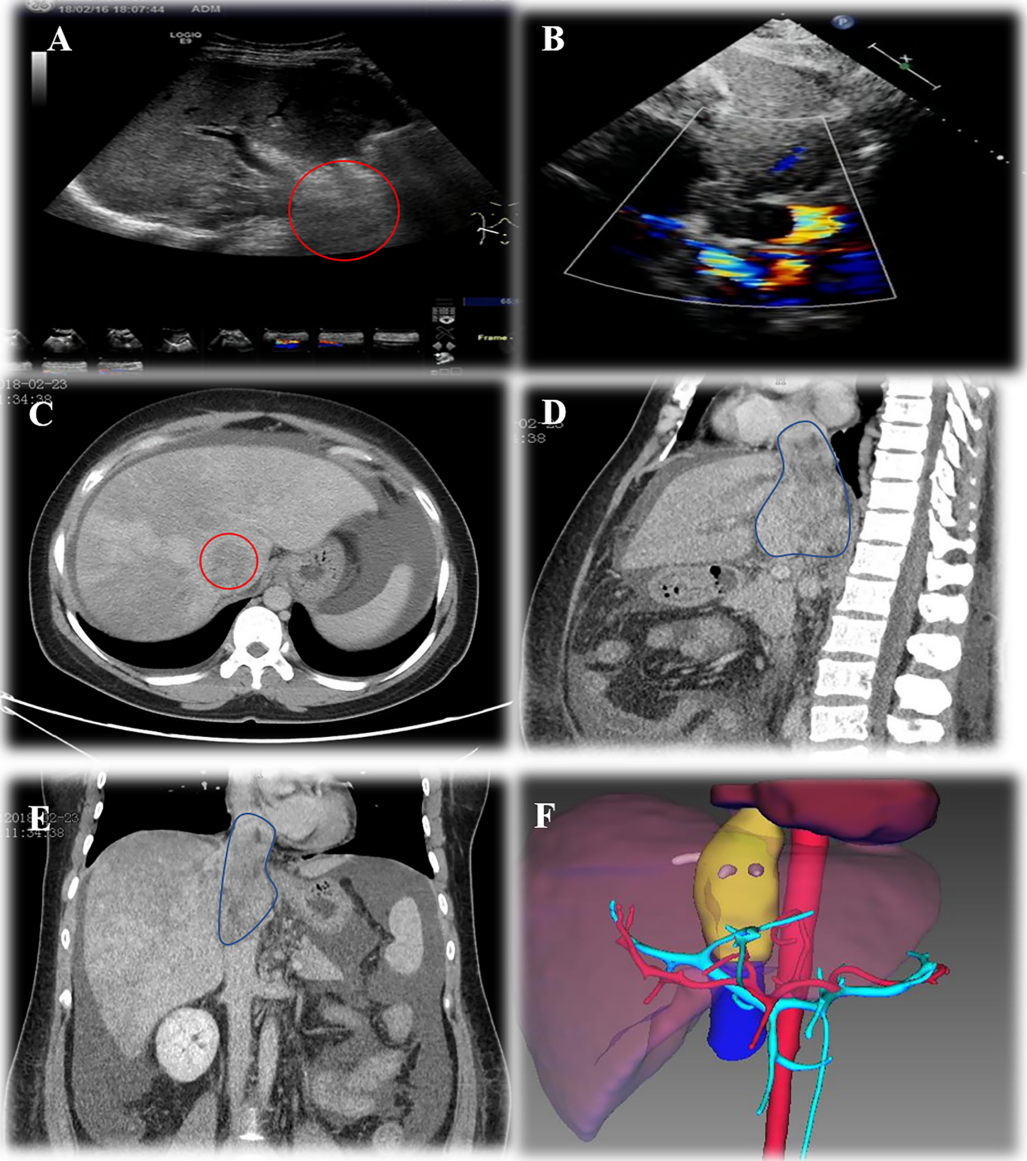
Preoperative assessment of Leiomyosarcoma. (A), ultrasonography showed haeato-caval mass; (B), echocardiogram showed lesion extending into right atrium; (C), cross section CT scan showed lesion in IVC; (D), sagittal section CT scan showed lesion extension and ascites; (E), coronal section CT scan of IVC lesion; (F), three-dimensional reconstruction showed lesion extension.
